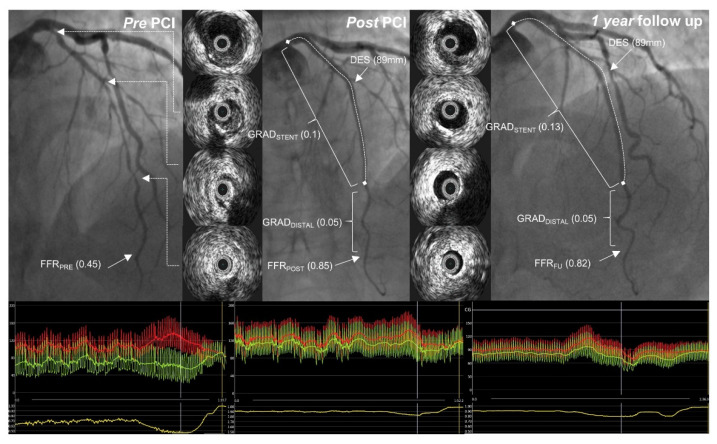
A case example. Pre-, post-percutaneous coronary intervention (PCI) and one-year follow-up angiographic images with intravascular ultrasound pictures from a corresponding left anterior descending artery segment. Fractional flow reserve curves are presented below. FFR_PRE_—FFR before PCI; FFR_POST_—FFR post-PCI; GRAD_DISTAL_—distal gradient (from the distal segment to the distal stent edge); GRAD_STENT_—trans-stent gradient; FFR_FU_—FFR recorded at follow-up.
